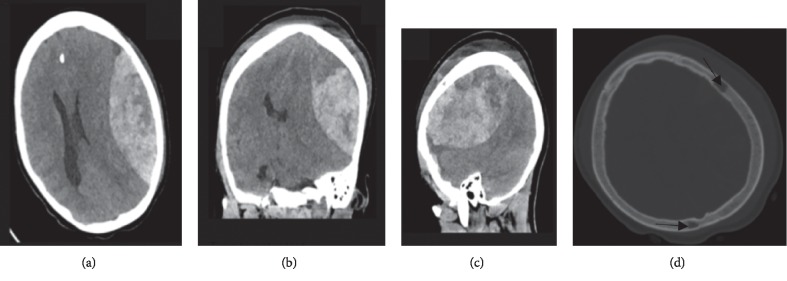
(a) Axial CT brain scan (no contrast) demonstrating left-sided extradural hematoma. (b and c) Coronal and sagittal CT brain scans, respectively, showing the extradural hematoma and subgaleal hemorrhage. (d) The preoperative CT on bone window showed two hypodense lesions (arrows) on the left frontal and right parietal bones.
